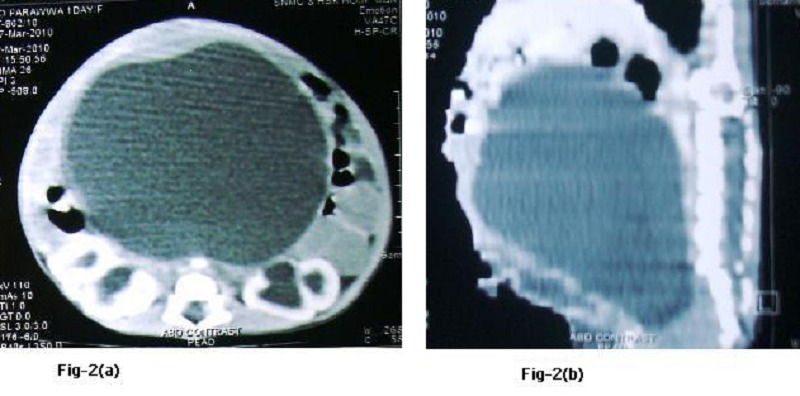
Figure 2 (a, b): CT images showing severe hydrometrocolpos.
